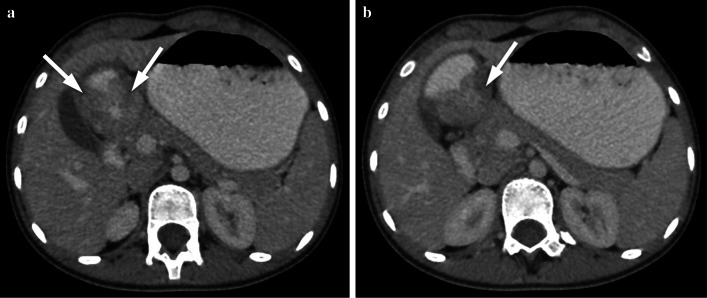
a, b A 12-year-old girl with gastric adenocarcinoma (signet ring cell carcinoma). She had complaints of abdominal pain, vomiting and weight loss that started 3 months ago. a, b Axial contrast-enhanced CT images demonstrate hypodense tumor at the antrum of the stomach (arrows) observed as pathological wall thickening
